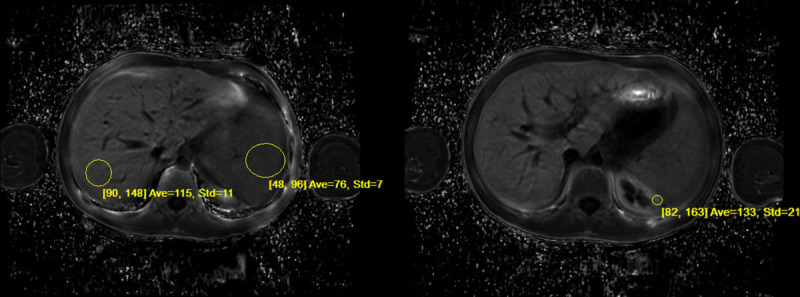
Ideal IQ R2* Sequence: MRI measurements estimating hepatic iron concentrations in the liver, spleen, and kidney using modified gradient echo sequences with the GE Discovery 450. Circled areas on image show areas used for calculating iron concentrations: Liver 3.1 mg per gram of liver tissue, spleen 2.4 mg per gram of spleen tissue, and renal 3.0 mg per gram of kidney tissue.
